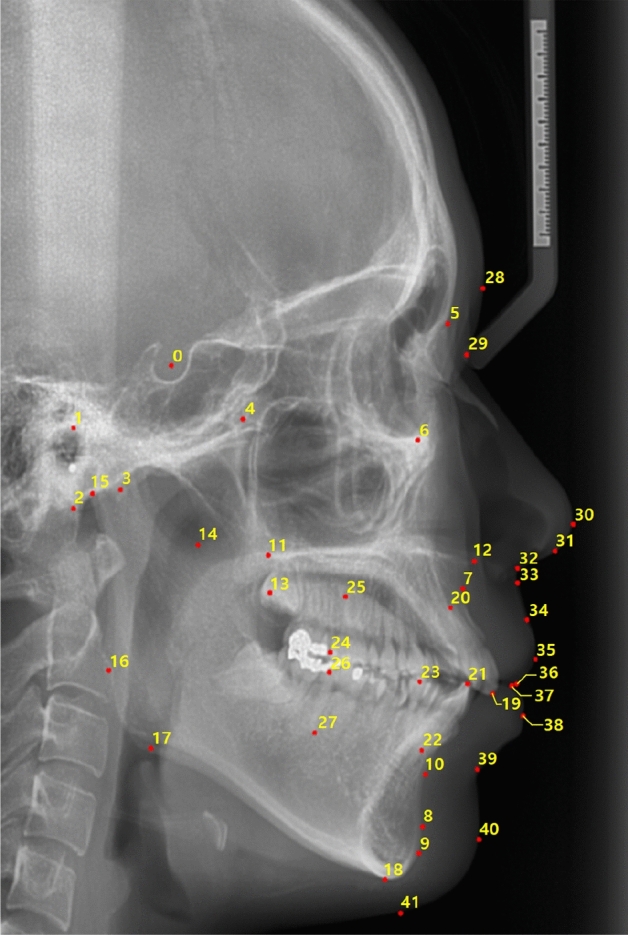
An example case of forty-two landmarks (numbered 0–41) in a cephalometric X-ray lateral image of size 2 k × 3 k pixel used in this study.
